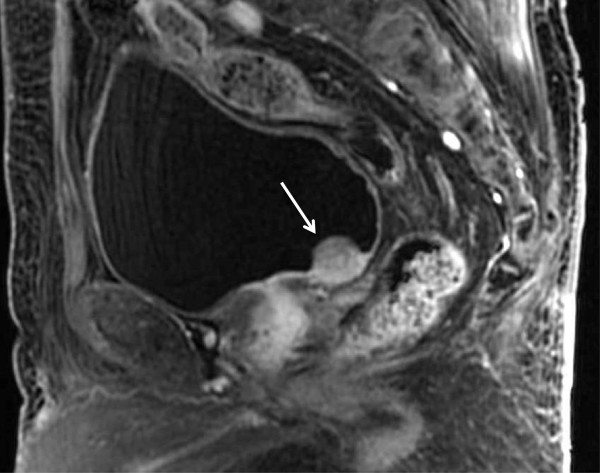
Contrast-enhanced magnetic resonance imaging (sagittal image) showed a bladder tumor (arrow) at the trigone, 20 × 17 × 15 mm in size, with muscle invasion.
